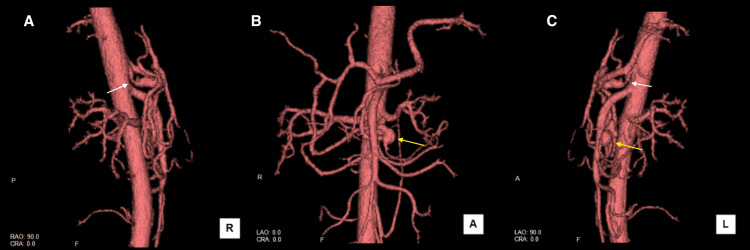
Three-dimensional computed tomography angiography: (A) right lateral view, (B) anterior view, and (C) left lateral view The celiac artery stenosis is indicated by the white arrows on the right lateral view (A) and left lateral view (C). The pancreaticoduodenal artery aneurysm is indicated by the yellow arrows on the anterior view (B) and left lateral view (C).
